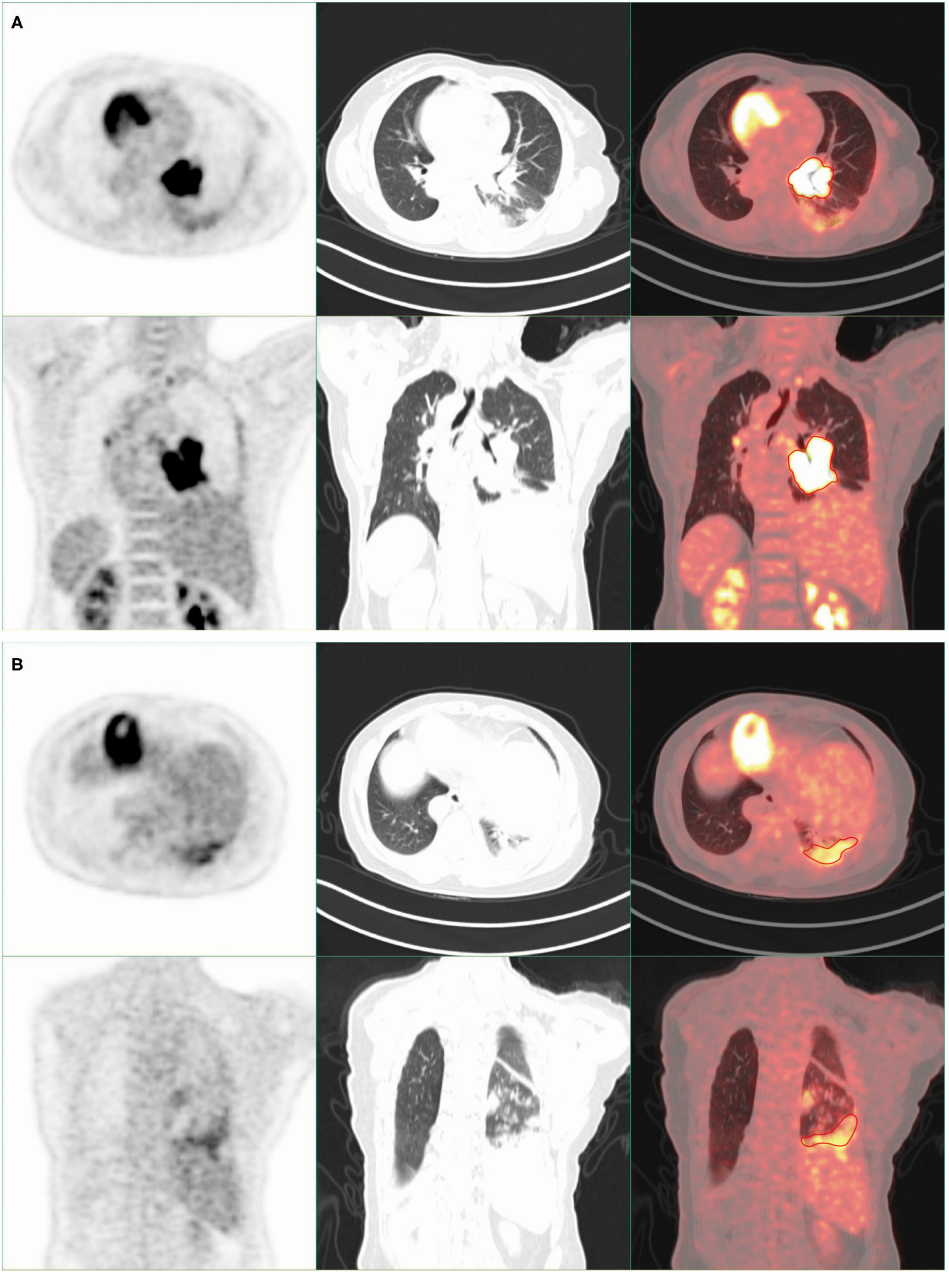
Chest imaging of positron emission tomography (PET)/CT scan. (A) There was high tracer uptake in the left hilar area. (B) The bronchus of the lower lobe of the left lung was truncated, and high tracer uptake was observed.
